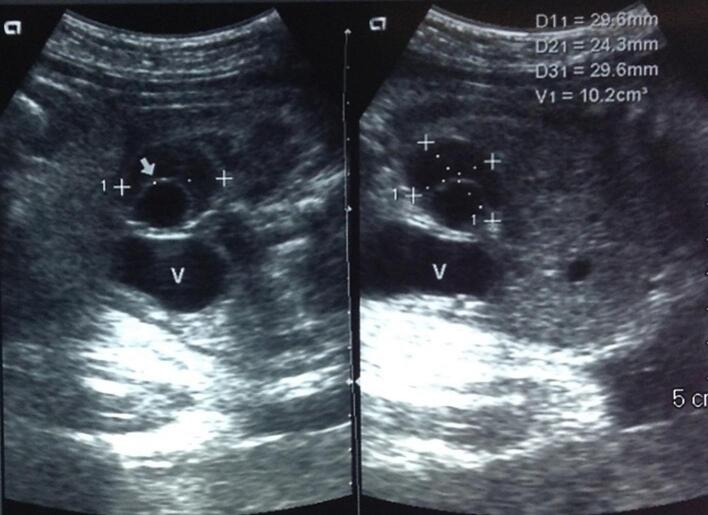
Abdominal ultrasound showing a right upper quadrant hypoechoic mass with an internal hyperechoic rim.
